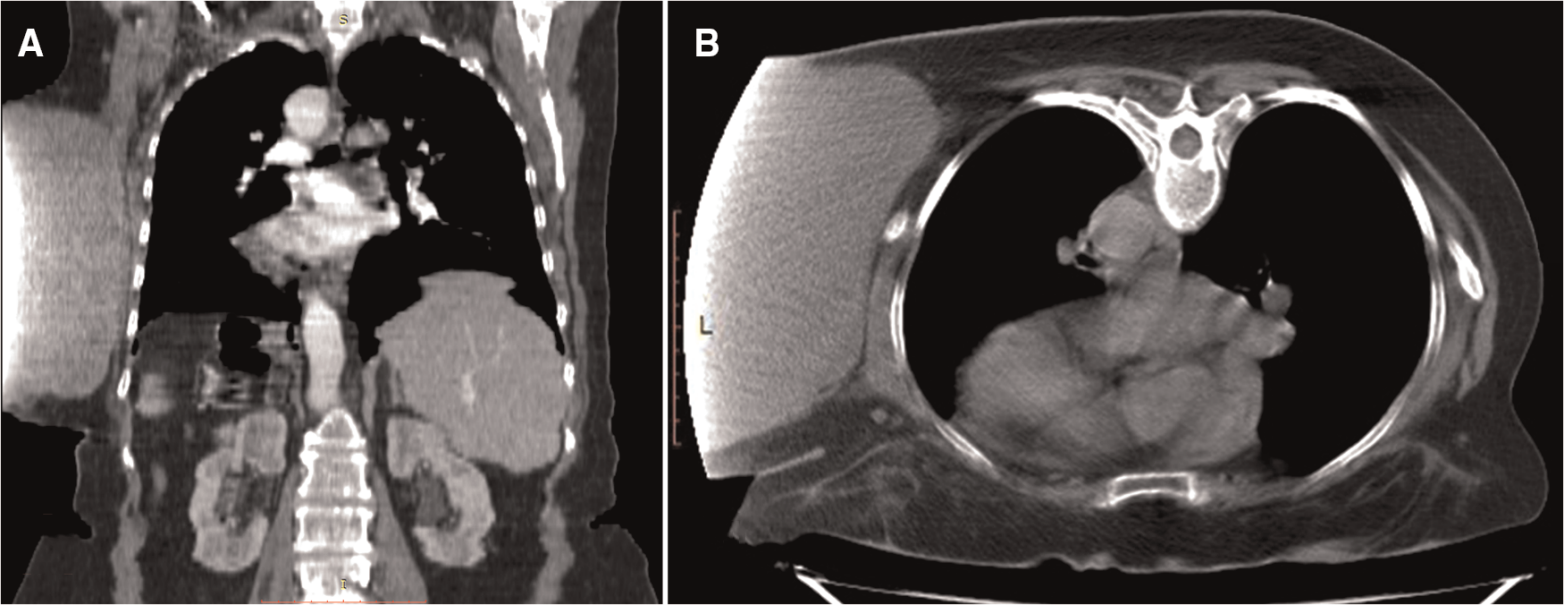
Chest soft tissue mass CT imaging. (А). Frontal view. (B). Axial view.
